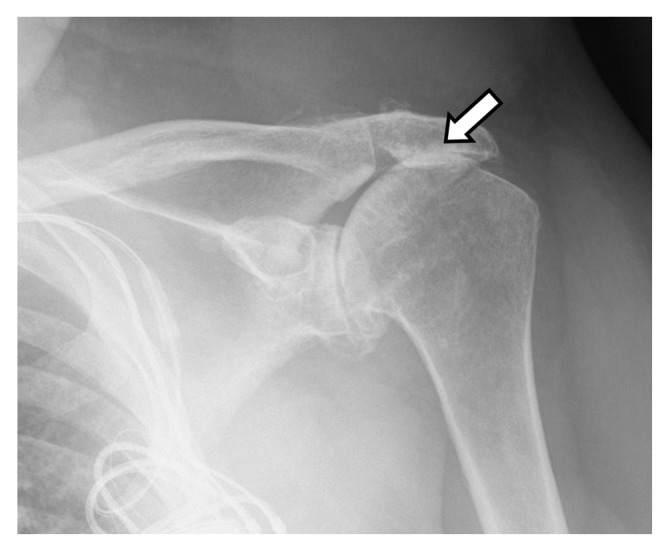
Frontal radiograph shows superior migration (white arrow) of the humeral head and developing acetabularization of the acromion in a patient with a full thickness rotator cuff tear.
